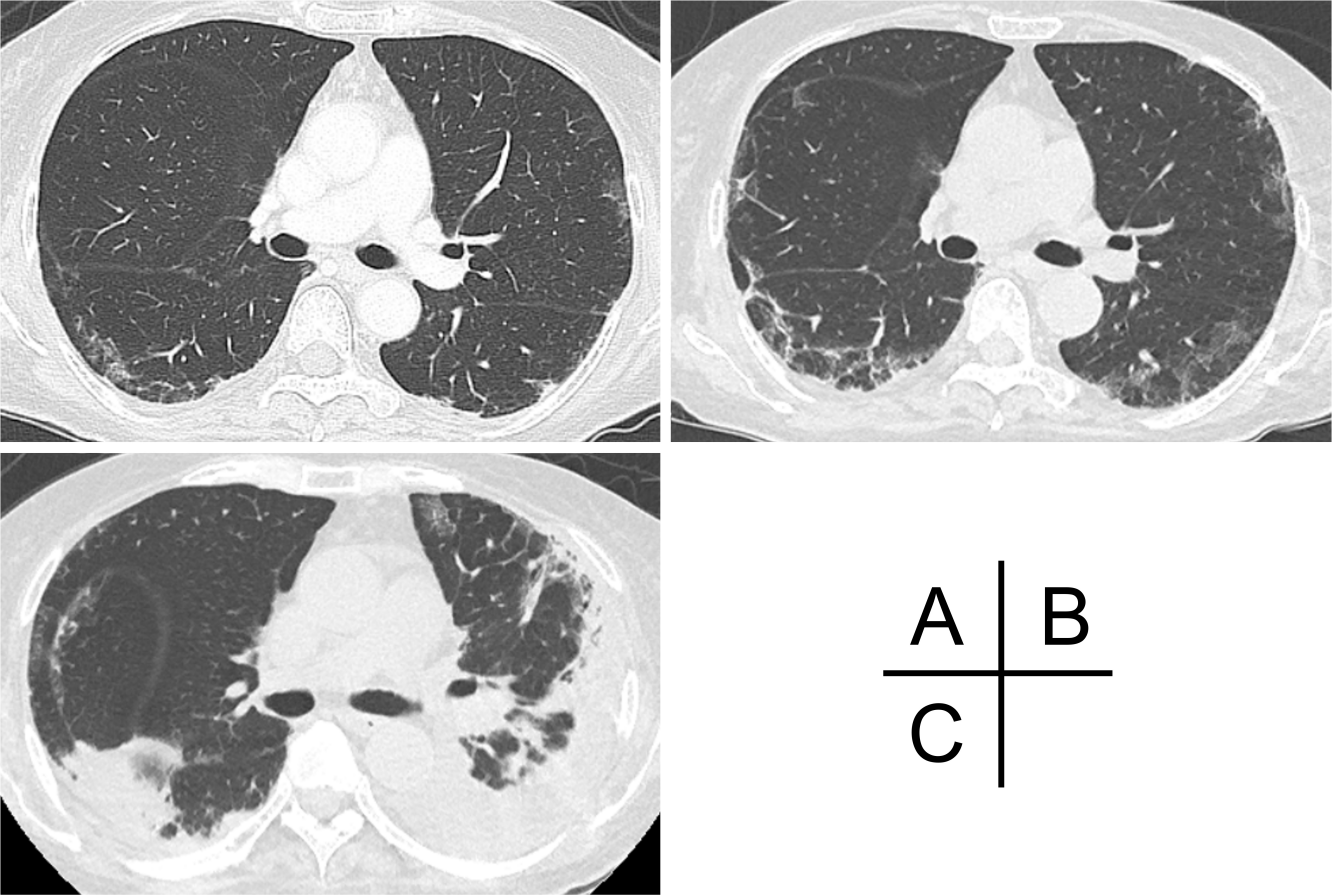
High-resolution computed tomography findings. (A) mild peripheral reticular shadows and GGOs in the bilateral lung lobes at the onset of spontaneous intramuscular hemorrhage. (B) Exacerbation of peripheral reticular shadows and GGOs in the bilateral lung lobes at the diagnosis of anti-MDA5 antibody-positive DM. (C) newly developed consolidation around the right interlobular pleura and increased pleural effusion in the left lung lobe along with worsening reticular shadows and GGOs in the bilateral lung lobes 1 month after remission induction therapy for anti-MDA5 antibody-positive DM. DM, dermatomyositis; GGO, ground-glass opacity; MDA5, melanoma differentiation-associated gene 5.
